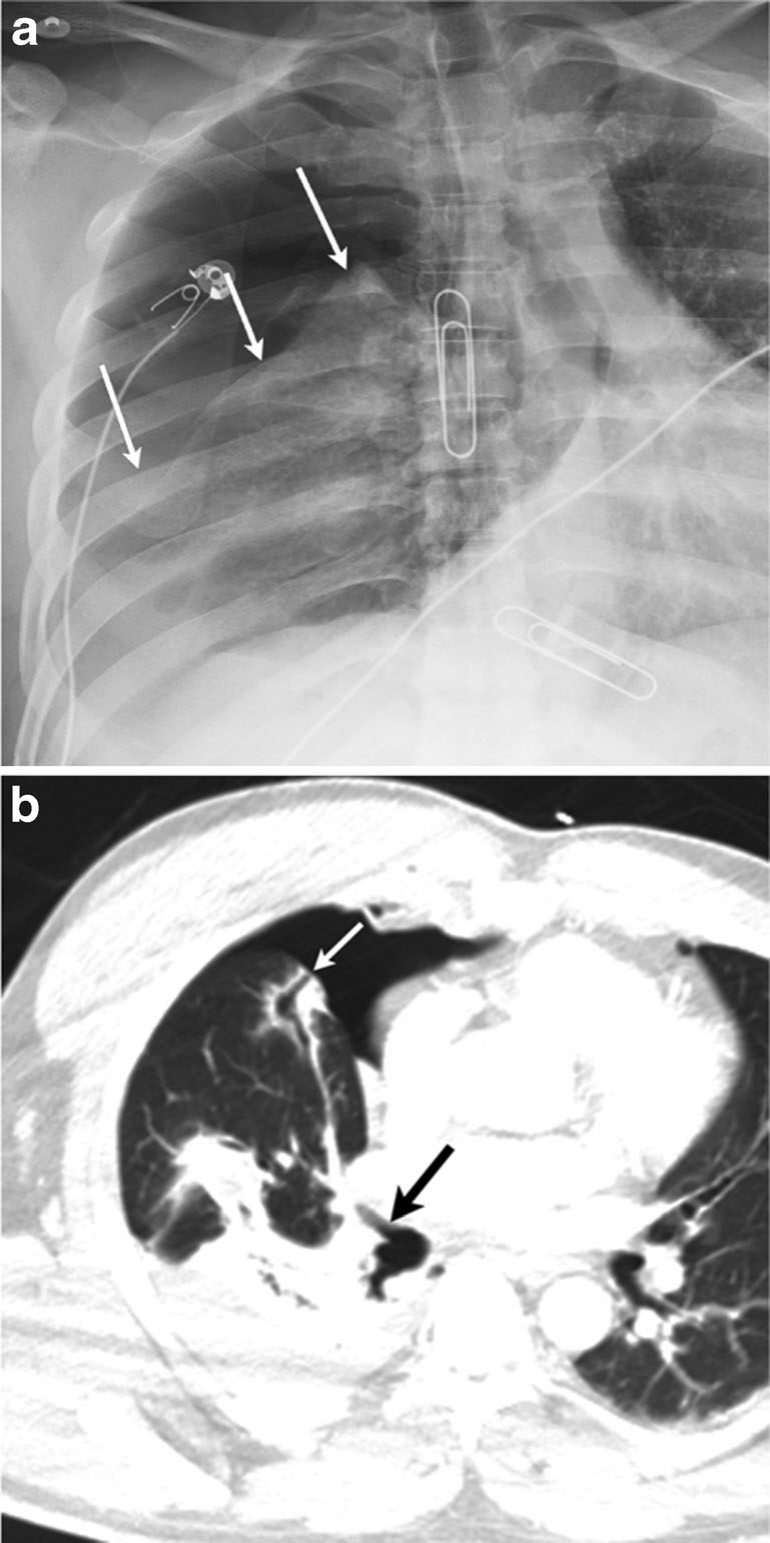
A 47-year-old male presented to the ED with a stab wound to the right chest. Frontal chest radiograph (a) demonstrates a right-sided tension pneumothorax (*arrows*). Axial image from CT (b) performed the same day demonstrated direct communication of the right middle lobe bronchus (*large arrow*) with the pleural space (*small arrow*)—a bronchopleural fistula
